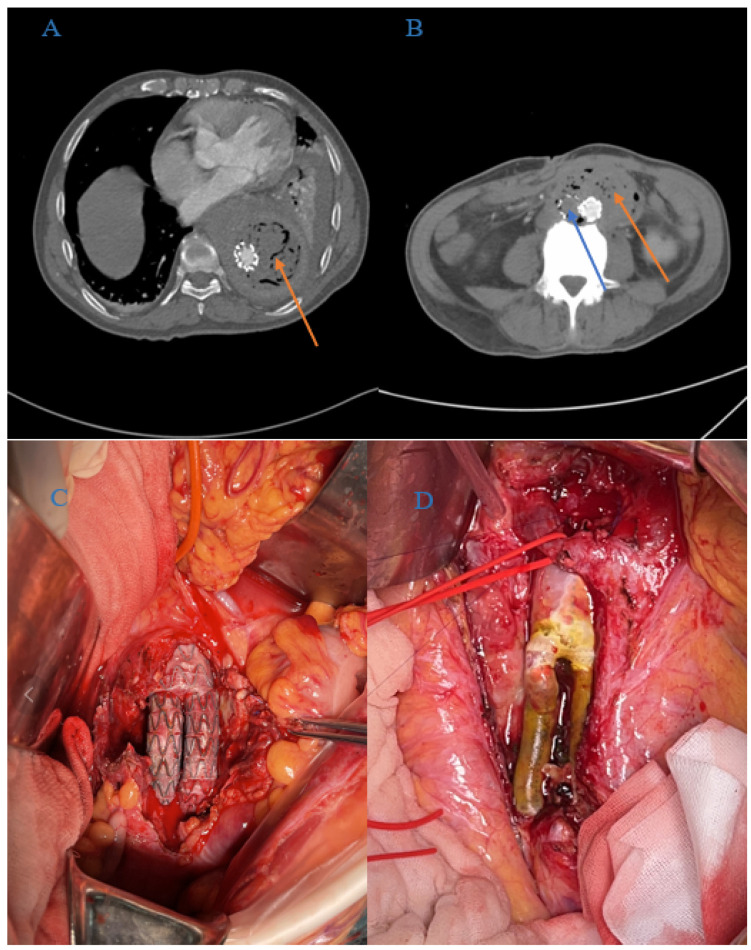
Peri-operative images of AGI patients. (A) Pre-operative CTA image of an AGI patient who previously underwent TEVAR (the orange arrow indicates the peri-graft fluid and gas). (B) Pre-operative CTA image of an AGI who underwent EVAR prior to AGI (orange and blue arrow indicate the peri-graft fluid and gas and graft thrombosis, respectively). (C) Peri-operative image of the ISR procedure. (D) Autologous vein was used as bypass graft conduits in ISR procedures.
